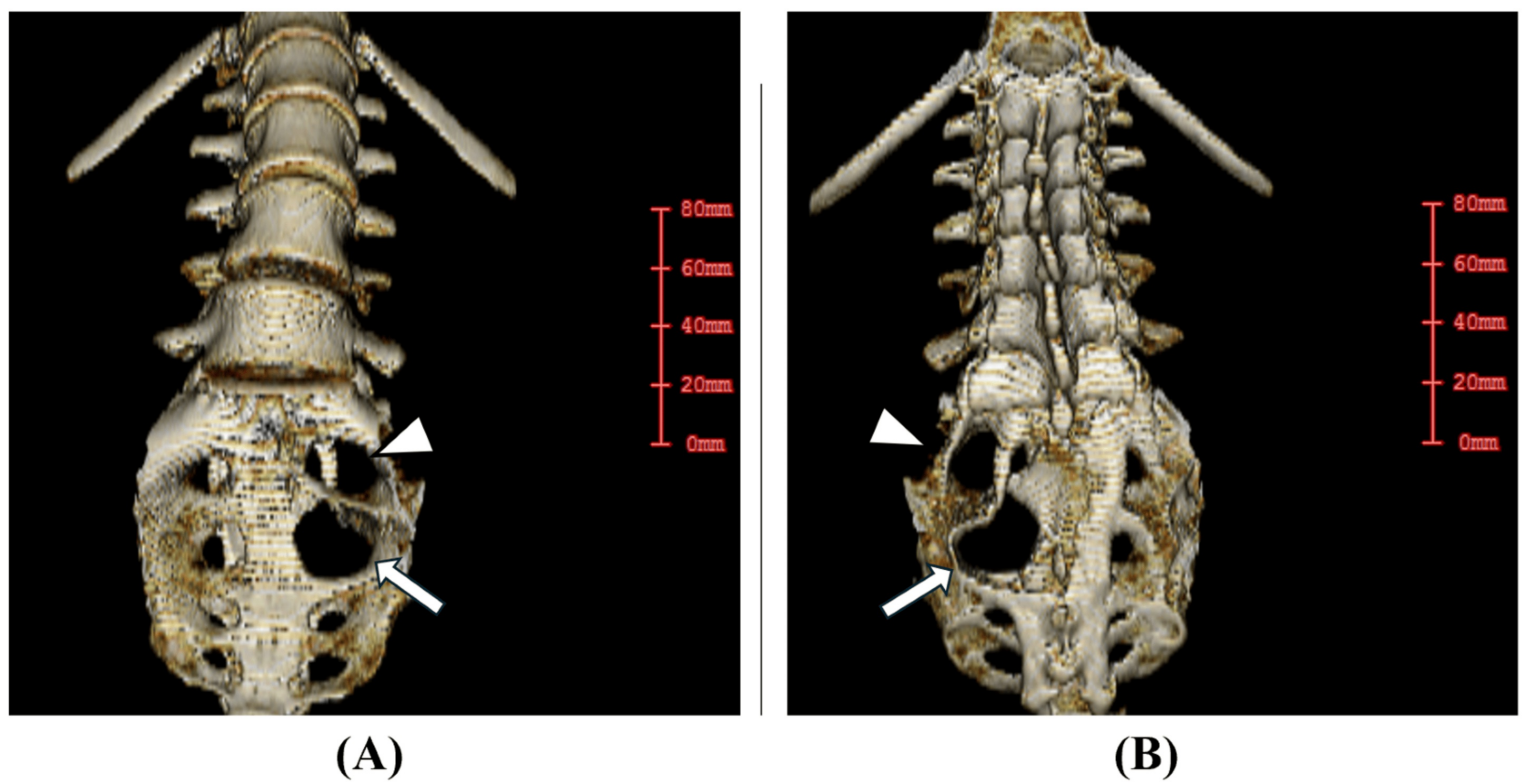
Initial pelvic CT Images (A) Lumbosacral 3D CT anterior view, (B) Lumbosacral 3D CT posterior view The cyst was large and associated with bone destruction of the left S2 foramen (arrowheads in A, B) and left S3 foramen (arrows in A, B).
